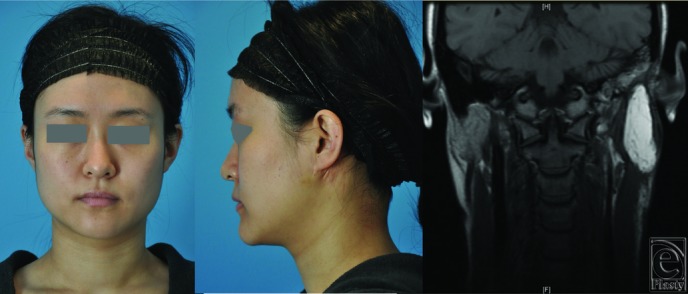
Postoperative images: (a) frontal view and (b) lateral view. Excellent facial symmetry is evident. (c) Postoperative magnetic resonance image demonstrating the superficial inferior epigastric artery flap within the parotidectomy bed.
